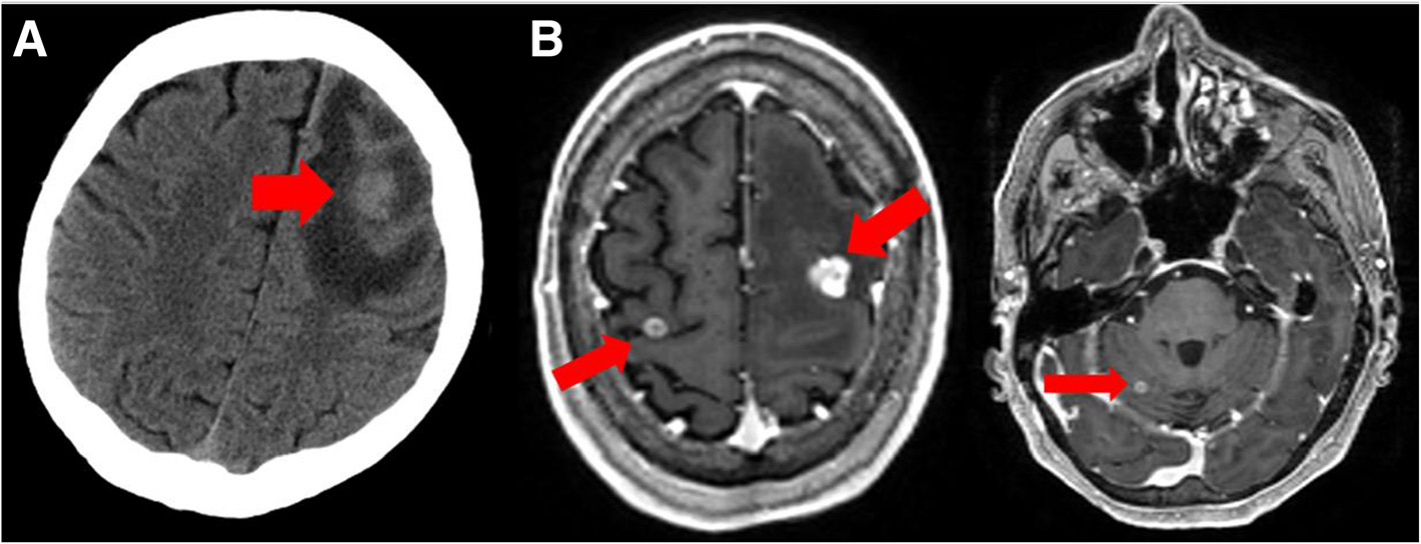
A patient known to have melanoma presents with a first seizure and (A) unenhanced CT brain (32-slice scanner, 10 mm slices) taken in the emergency department shows an abnormality in the left frontal lobe with surrounding oedema – they were referred for possible neurosurgical intervention. (B) T1 weighted MRI at 1.5 T with single dose (0.1 mmol/kg) gadolinium contrast detects this lesion but also delineates it further, allowing volume to be assessed accurately and in addition highlights two further areas of abnormality. After staging of the systemic disease and discussion with oncologists and surgeons, the patient was therefore treated with stereotactic radiosurgery to all three areas.
